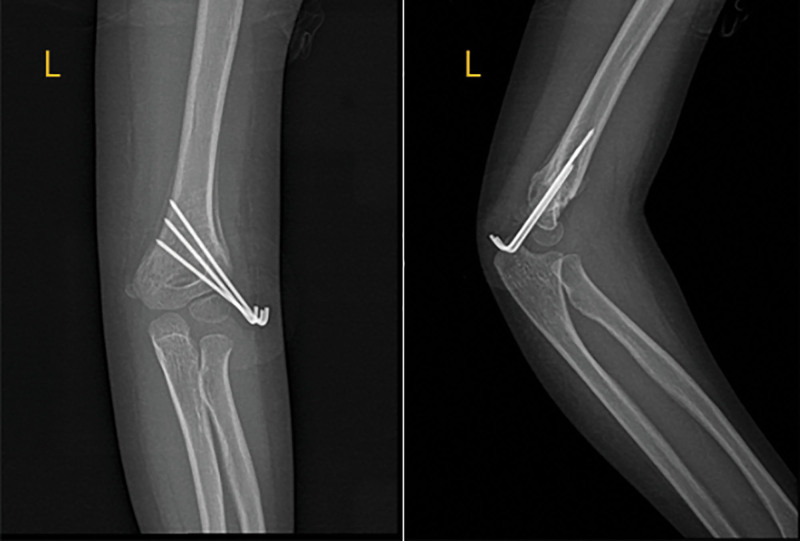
Elbow X-ray after CRPP surgery. CRPP = closed reduction and percutaneous pinning.
